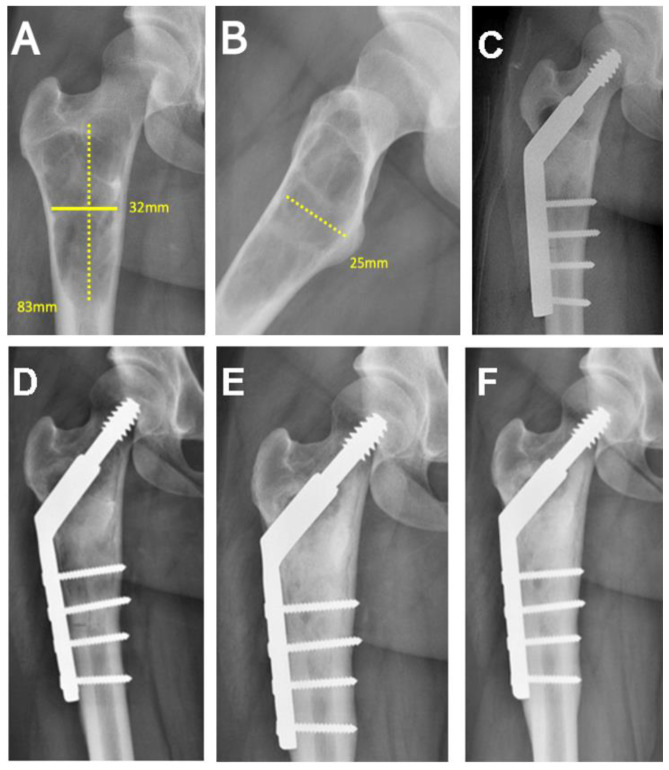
(A,B) Preoperative radiograph, anteroposterior, and lateral views. Volume measurement in a unicameral bone cyst of the proximal femur. Application of the volume formula ABC × 0.785 = 52.25 cm^3^ (cylindrical defect). (C) Immediate postoperative radiograph after curettage, grafting, and plate fixation. Bone graft homogeneously distributed in the cavity with >90% filling. (D) Postoperative 6-month follow-up radiograph showing cortical thickening and partial graft incorporation. (E,F) Radiographs at 12- and 24-month follow-up, showing complete graft incorporation (Neer I) and bone remodeling.
